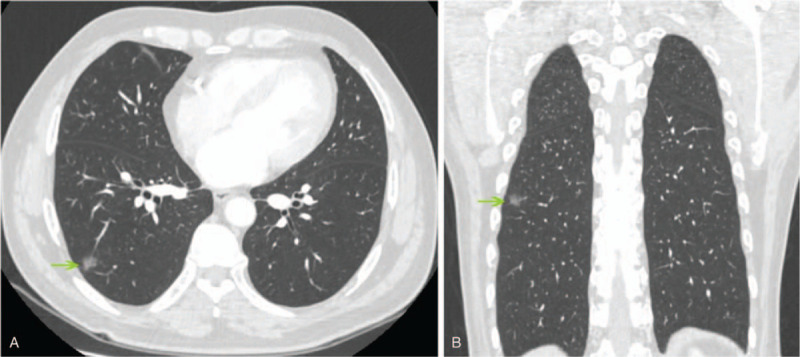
Subpleural 1.2-cm ground-glass opacity lesion (arrow) in the superior segment of the right lung lower lobe observed on chest CT imaging, with axial (A) and coronal (B) images. CT = computed tomography.
